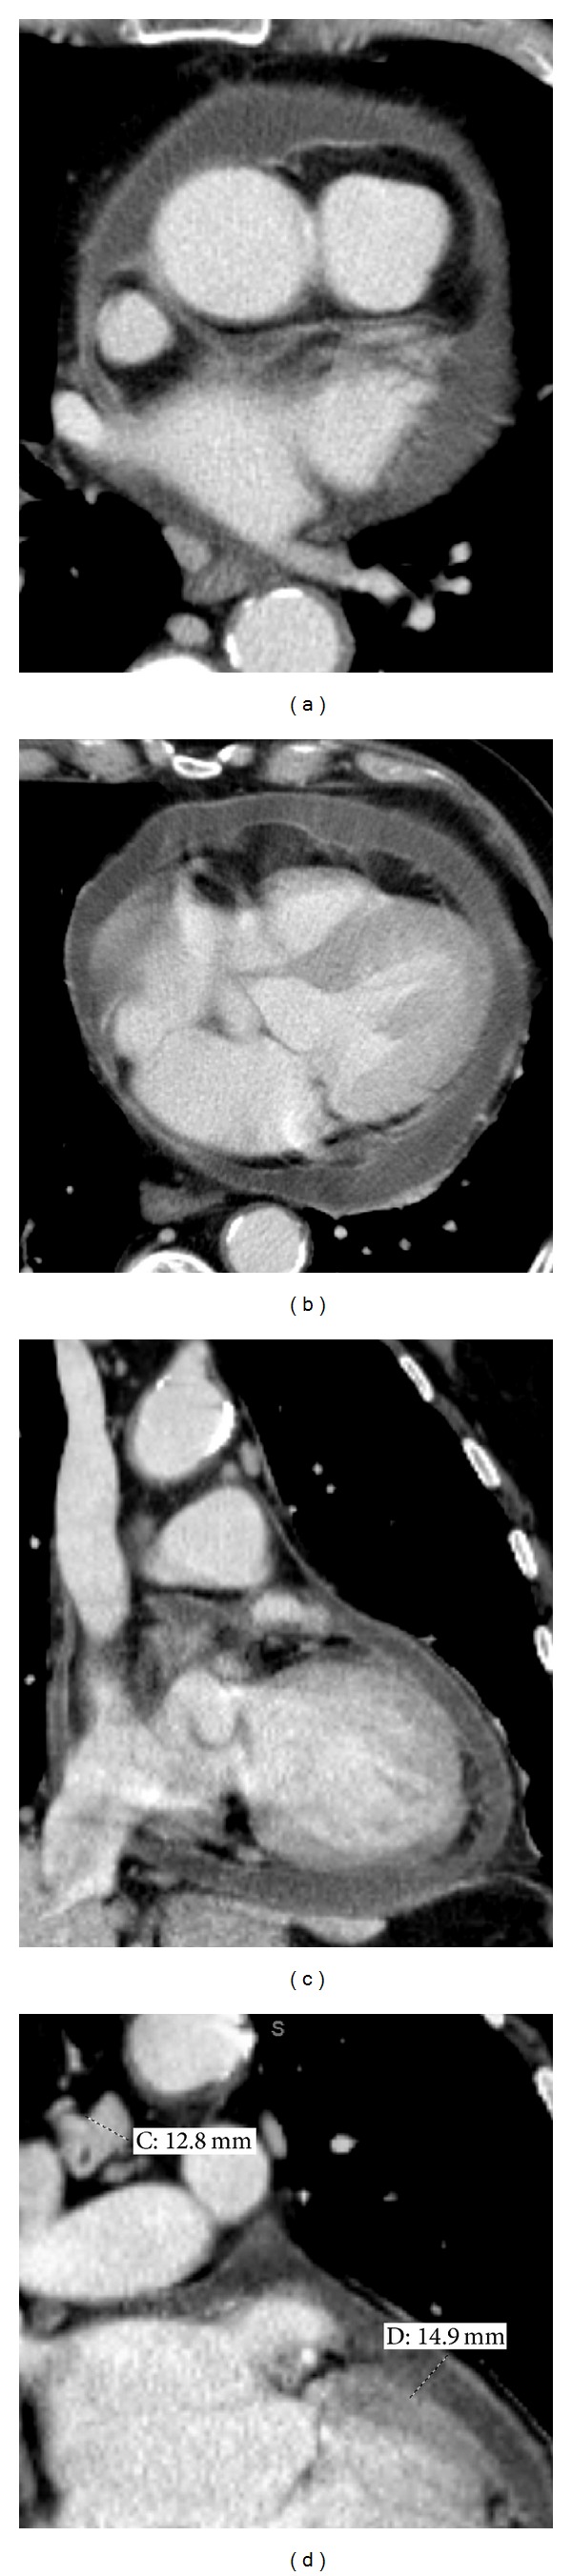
Idiopathic/viral pericarditis. An 83-year-old male with history of coronary artery disease and hypertension presents to the emergency department with a 5-week history of fever and malaise. CECT performed for fever of unknown origin demonstrates pericardial enhancement and effusion (a–c), mediastinal lymphadenopathy (d), and a solid enhancing right renal mass (not shown). No cause for the patient's pericarditis was found. Aspiration yielded occasional lymphocytes. Culture was negative. Fine needle aspiration of a mediastinal lymph node showed reactive cells. Symptoms gradually resolved on aspirin 325 mg daily. The patient's incidentally discovered that renal cell carcinoma proved to be nonmetastatic by PET-CT which was performed later.
